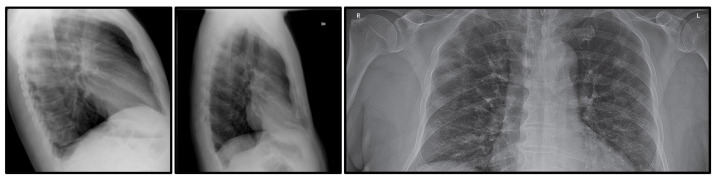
Samples of removed X-ray images. The first two samples are removed because they were captured from the side view, while the third sample is removed because of the incomplete information on the frontal chest X-ray image.
